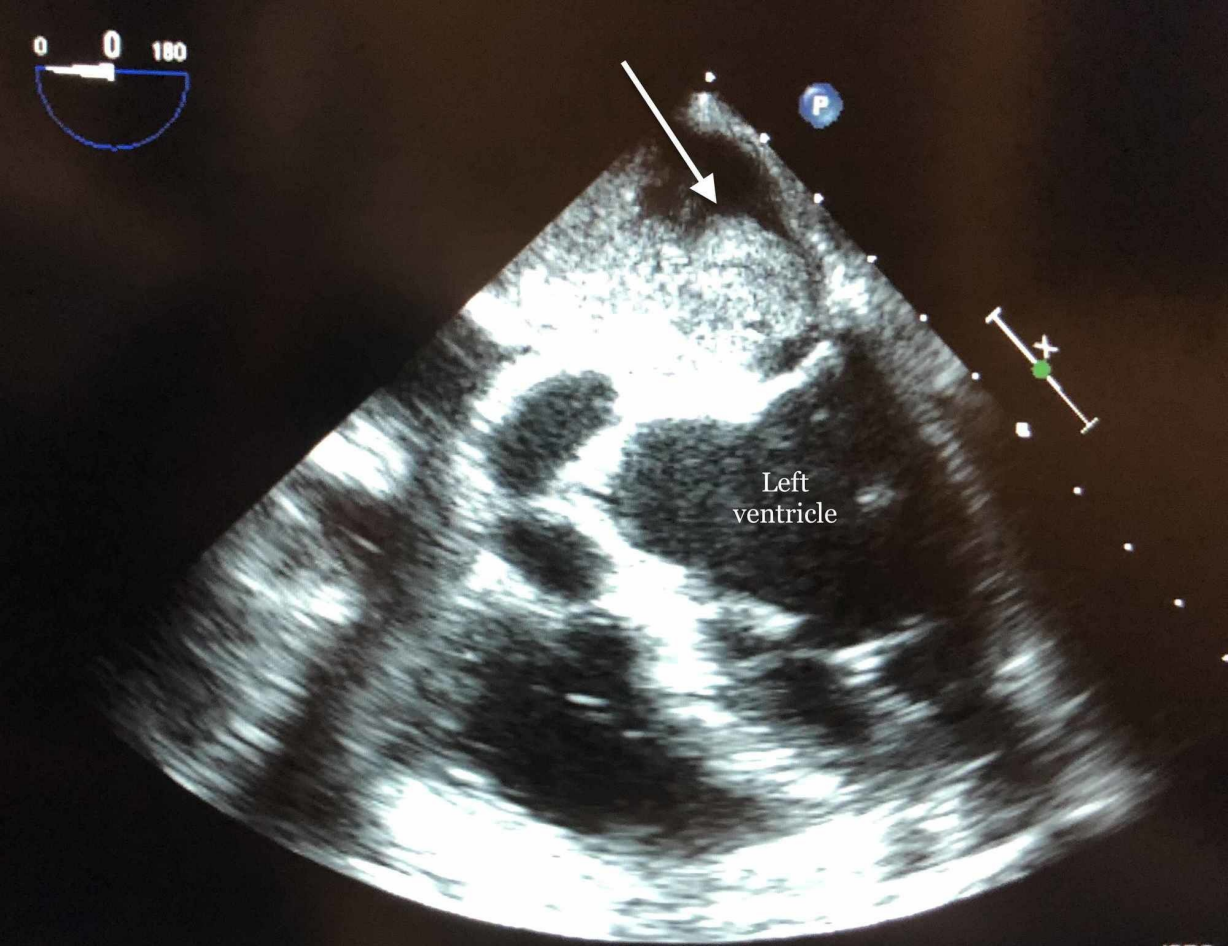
Transesophageal echocardiogram depicting left atrial myxoma (white arrow).
